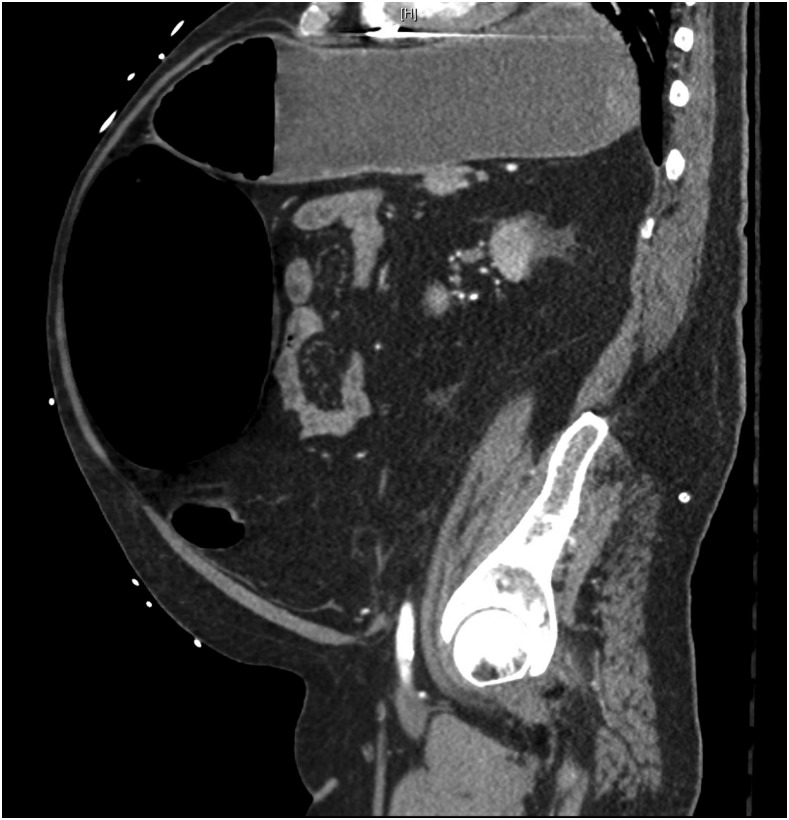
Computed tomographic study of the abdomen and pelvis in the sagittal plane revealing distention of the stomach, duodenum, and transverse colon.
